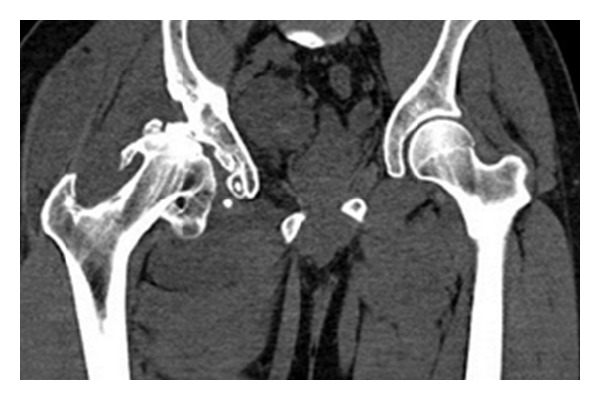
The CT scan coronal cut demonstrates numerous osteophytes around the femoral head and acetabulum.
